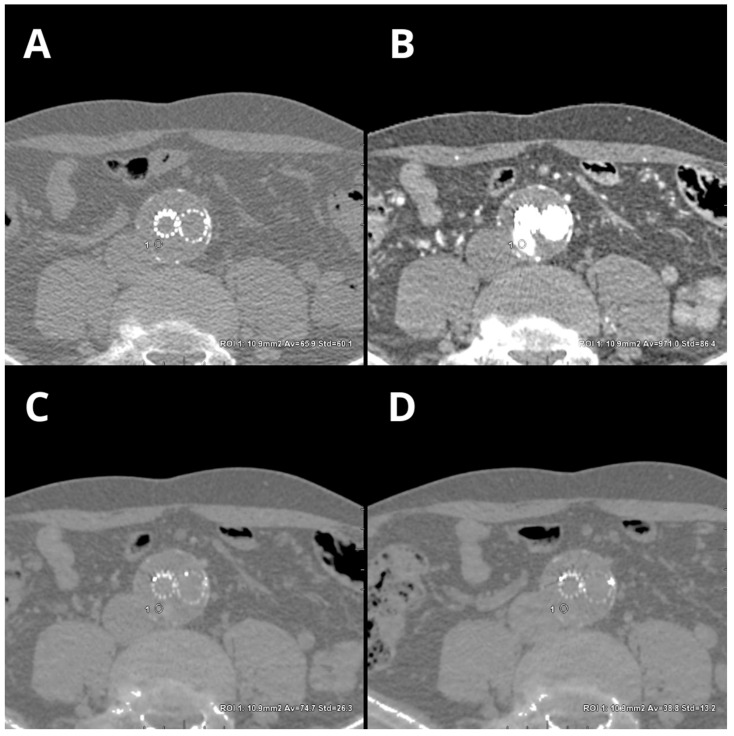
Differences in CT attenuation (average density ± SD) in small endoleak cases with true non-contrast, 40 keV VMI (arterial phase), and two VNC phases. Reconstructions: TNC ((A)—65.9 ± 60.1 HU), 40 keV VMI ((B)—971 ± 86.4), VNC arterial ((C)—74.7 ± 26.3 HU), and VNC delayed ((D)—38.8 ± 13.2 HU). An automatic region-of-interest (ROI) propagation tool was used with the same window settings (W 500, L 100).
